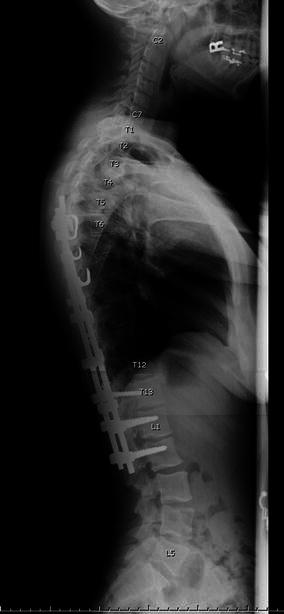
Lateral radiograph of the same patient as in Fig. 1 confirms the extra thoracic level is present
